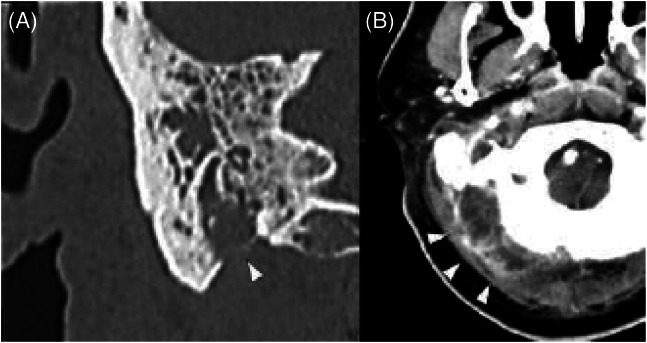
(A) Computed tomography (CT) of the mastoid with intravenous (IV) contrast showing mastoid process perforation (arrowhead); (B) CT of the neck with IV contrast demonstrating a posterior neck abscess (arrowheads).
